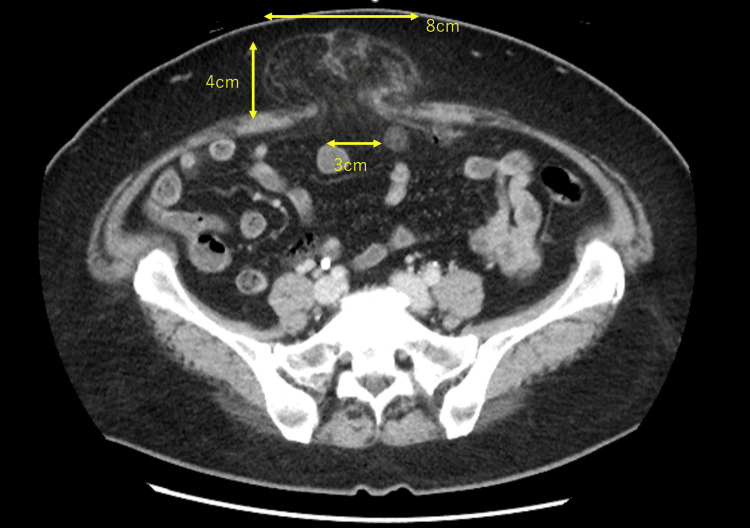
Computed tomography confirmed an umbilical hernia, with the hernia contents suspected to be the omentum.
